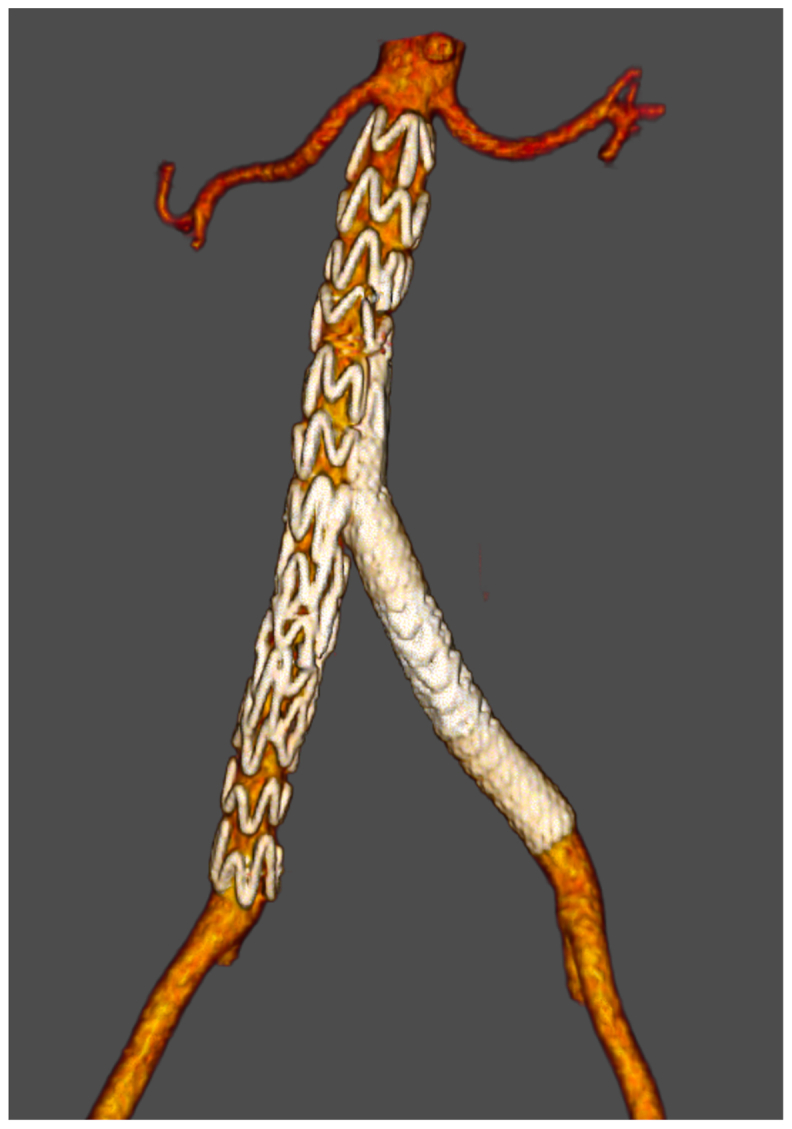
Thirty-day follow-up computed tomography (CT) angiography with three-dimensional reconstruction demonstrating complete aortic remodeling, preserved endograft patency, and restoration of normal aortoiliac anatomy.
